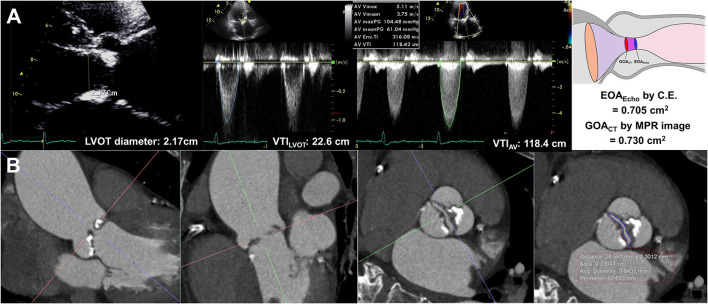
Effective orifice area by continuity equation (A) and geometric orifice area by multiplanar computed tomography image (B).
